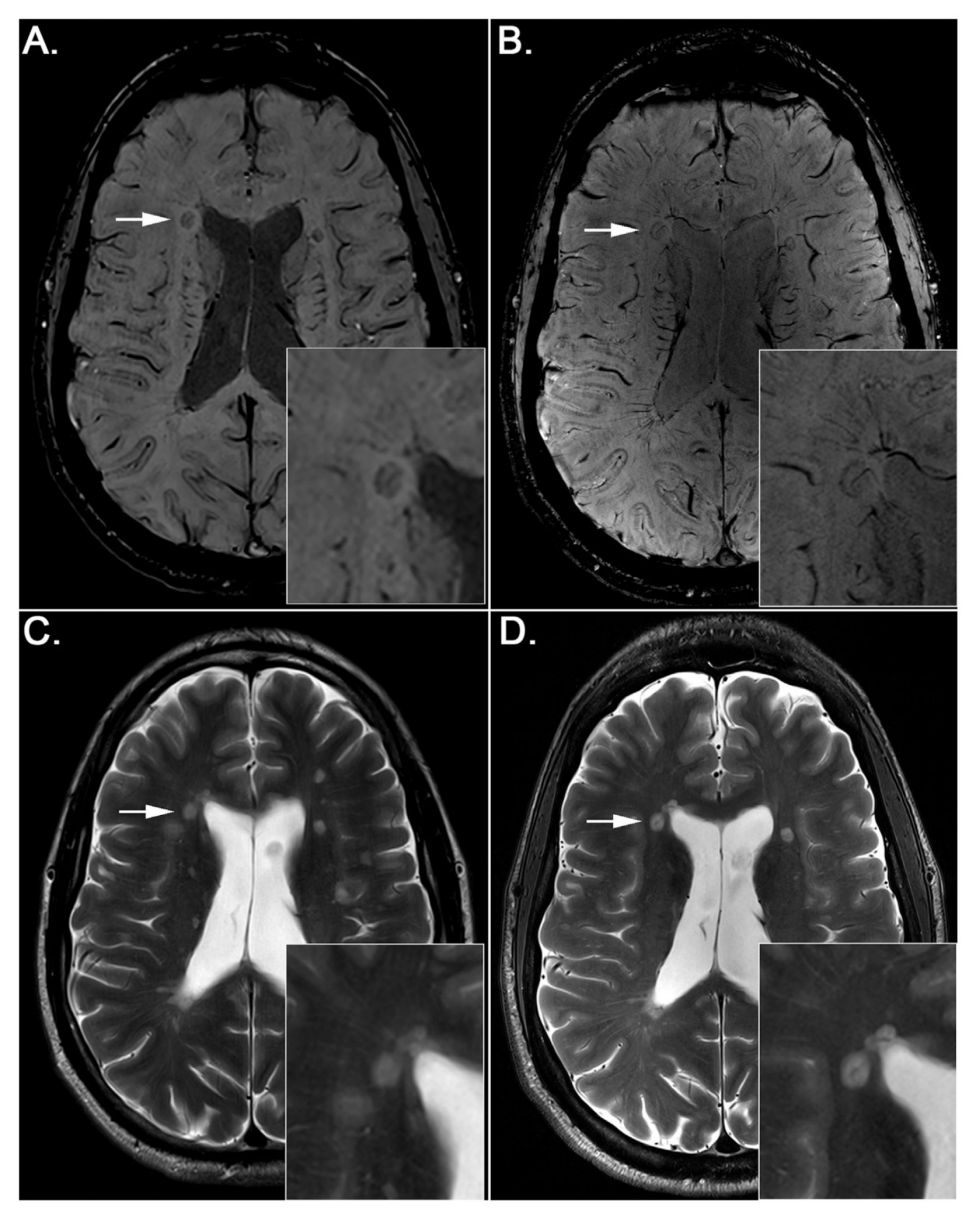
Axial SWI images of an MS patient acquired at 3 T (A) and 7 T (B) and corresponding T2-weighted images acquired at 3 T (C) and 7 T (D). Note iron deposits (rims) and central vessels within MS lesions in SWI images (A, B) (white arrows). In T2-weighted images central vessels are difficult to detect (C, D) (white arrows).
